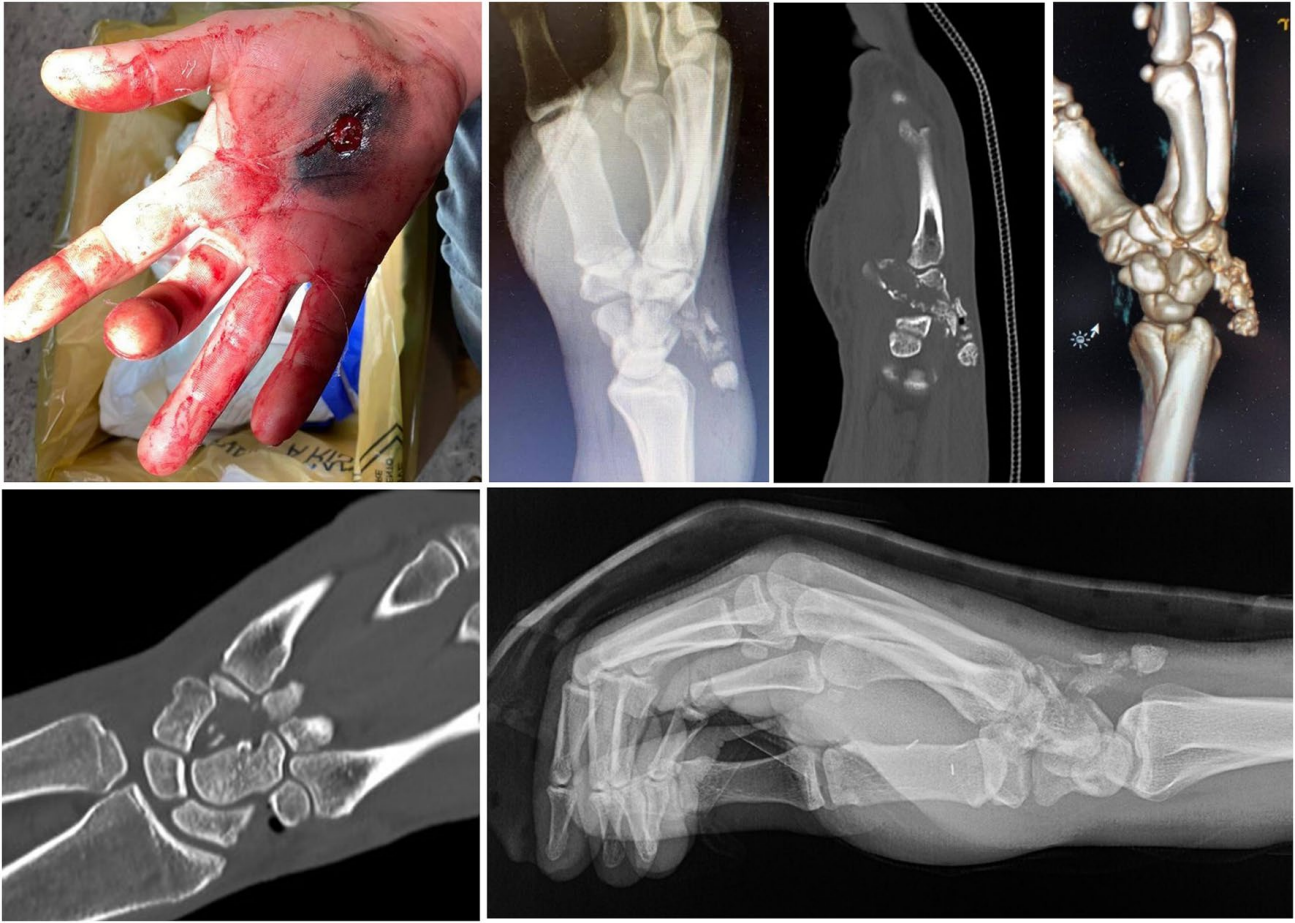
Penetrating injury of the right hand. X-ray scan and TC showed hamate bone comminute fracture and dislocation in the subcutaneous plane. After the first procedure in which debridement and nervous and tendinous reconstruction was performed, a plaster cast was applied
